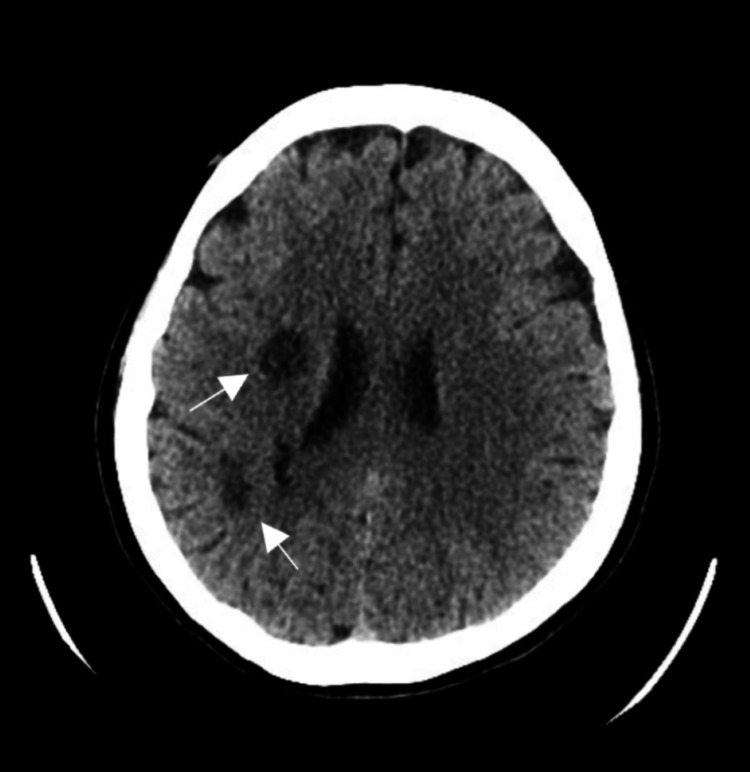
Plain CT brain showed multiple well-defined hypodensities in the right fronto-temporo-parietal regions, suggestive of subacute or chronic infarcts.
